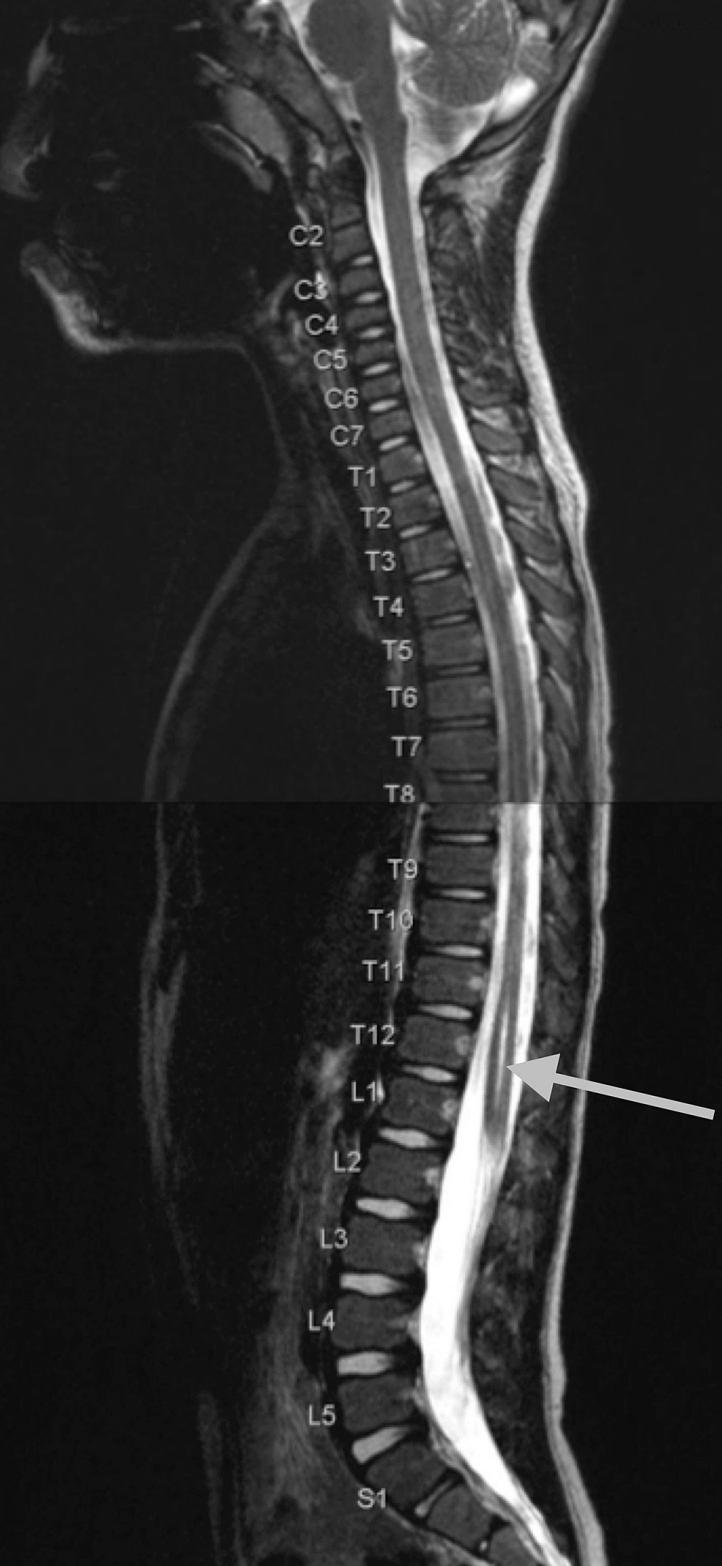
Stitched sagittal T2-weighted images of the cervical, thoracic, and lumbar spine numbered by level at five years of age show a slit-like syrinx from the conus medullaris and tapering cranially to C6-7, which is most prominent in the lower cord (gray arrow).
